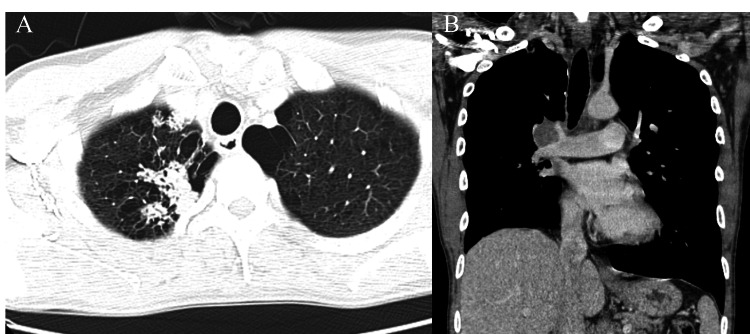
Recurrence sites on computed tomography At the time of first recurrence (26 months after surgery). (A) Multiple lesions in the right upper lobe, (B) Right hilar lymph node.
